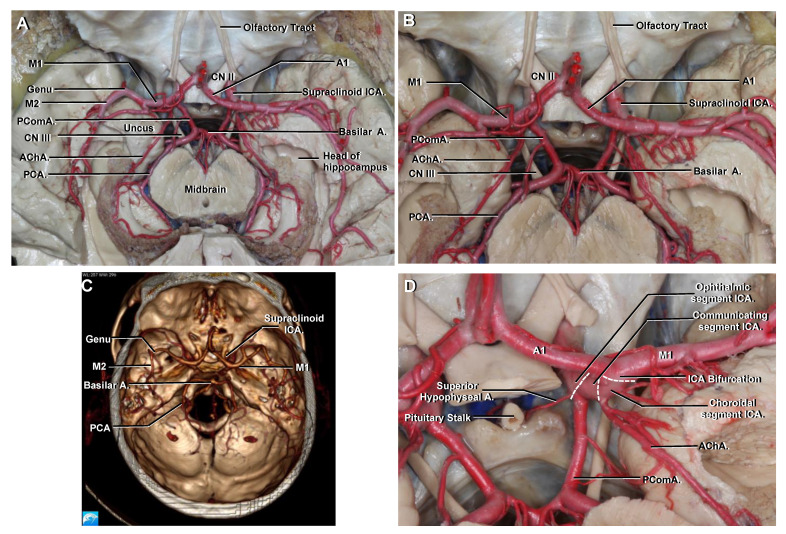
Superior view to demonstrate the supraclinoid ICA, circle of Willis and adjacent structures as seen in an axial CTA. (A) The circle of Willis lies above the sella turcica and surrounds the infundibular stalk. The apex of the uncus of the temporal lobe is seen pointing towards the oculomotor nerve. The PComA arises from the posterior surface of the communicating ICA and connects the anterior with the posterior circulation. The AChA also arises from the posterior surface of the ICA and is intimately related to the uncus of the temporal lobe. (B) Enlarged view from A. The PComA lies superomedial to the oculomotor nerve and has a roughly parallel trajectory to it. (C) Axial CTA VR reconstruction to be correlated with A and B. It is possible to identify the main intracranial arteries but not small branches like the AChA or PComA. (D) Enlarged view seen from the left. The three segments of the supraclinoid ICA are seen. The AChA arises a few millimeters distal to the PComA origin. Abbreviations: CTA., computed tomographic angiography; PComA., posterior communicating artery; VR., volume-rendered; AChA., anterior choroidal artery; PCA., posterior cerebral artery. See Figure 1, Figure 2 and Figure 3 for other abbreviations.
